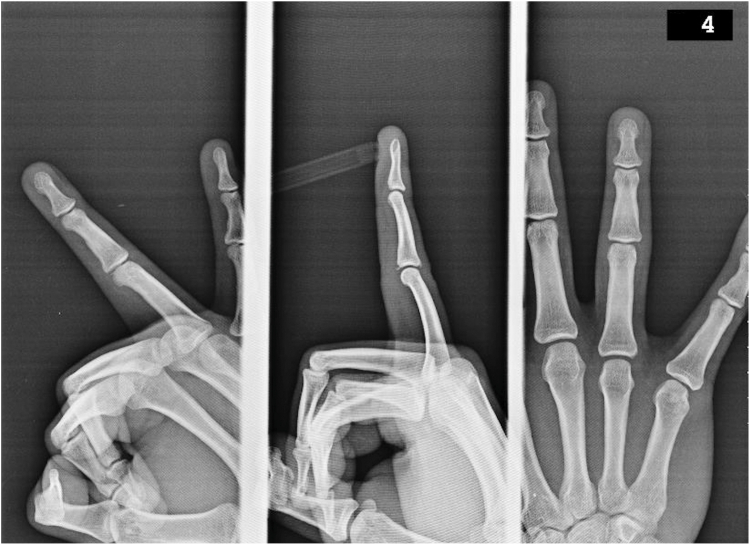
Radiograph demonstrating dorsal avulsion fracture (central slip) and volar fragment (volar plate) of the same finger.
